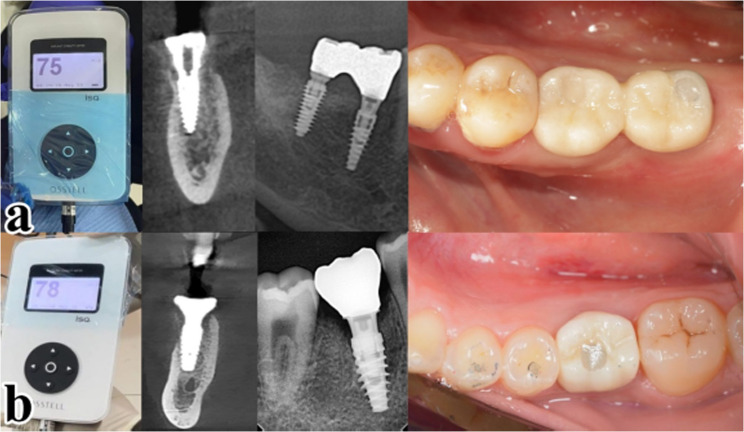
Display post operative secondary stability measure with Ostell, CBCT coronal cut, and periapical xray after 3 month and clinical pictures for screw retained crowns
